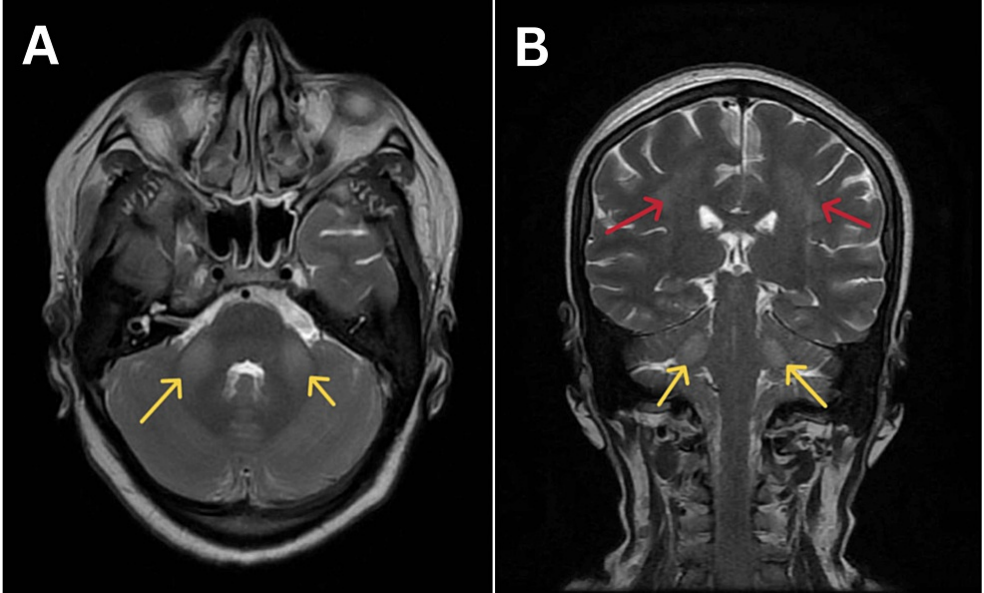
Magnetic resonance imaging of the brain of the patient suggestive of flair sequences showing hyperintensities in the middle cerebral peduncle (yellow arrows) and hyperintensities in parietal lobes (red arrows); A) axial section B) coronal section
